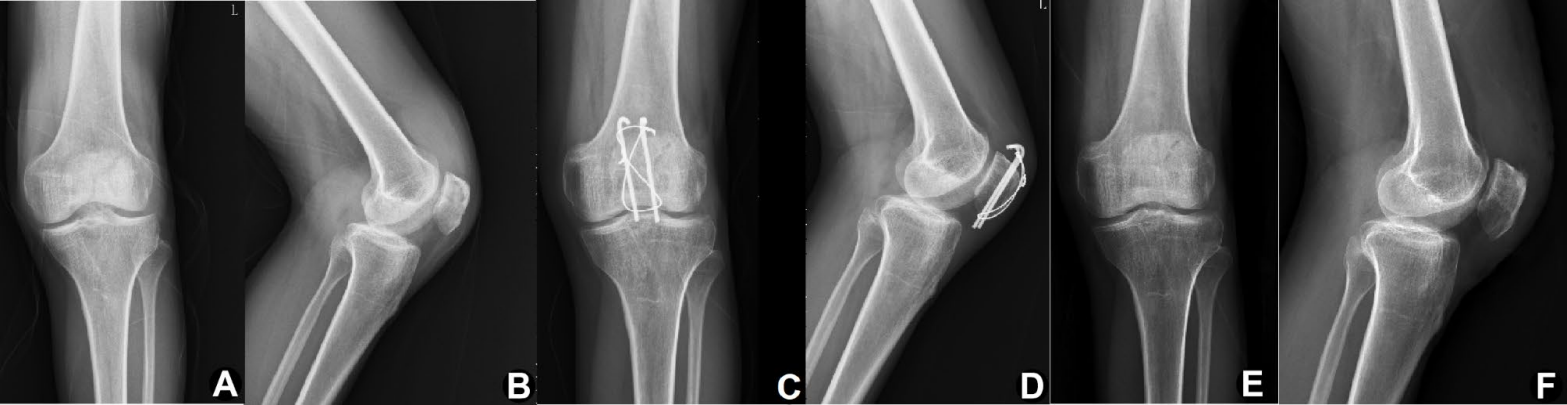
Case example of comminuted patellar fracture (male, 51 years old, left side) using the suture reduction combined with the modified K-wire tension band. (A, B) Preoperative X-ray and (C, D) CT images indicated displaced comminuted patellar fractures. (E, F) The regular follow-up at 1.5 years after operation showed the fracture healed without fixation failure
